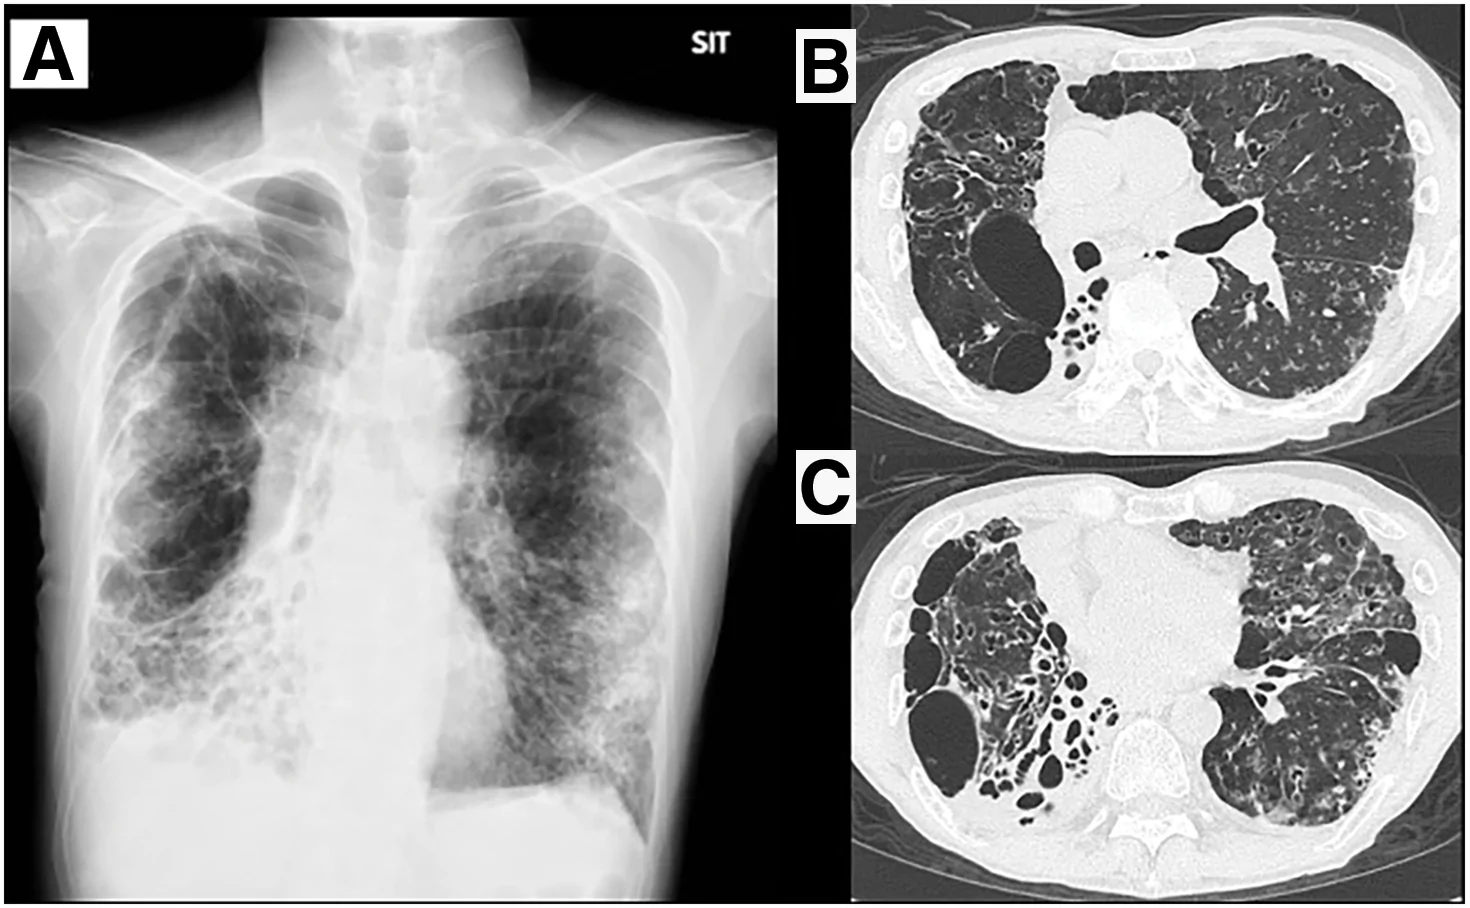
Chest radiograph (A) and CT (B, C) showing bilateral reticular opacities, centrilobular nodules, bronchiectasis, and bronchial wall thickening consistent with diffuse panbronchiolitis.
